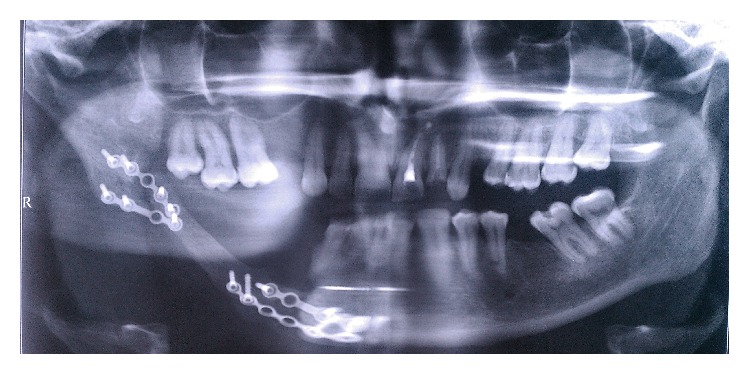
Panoramic radiography, next day after surgery.
